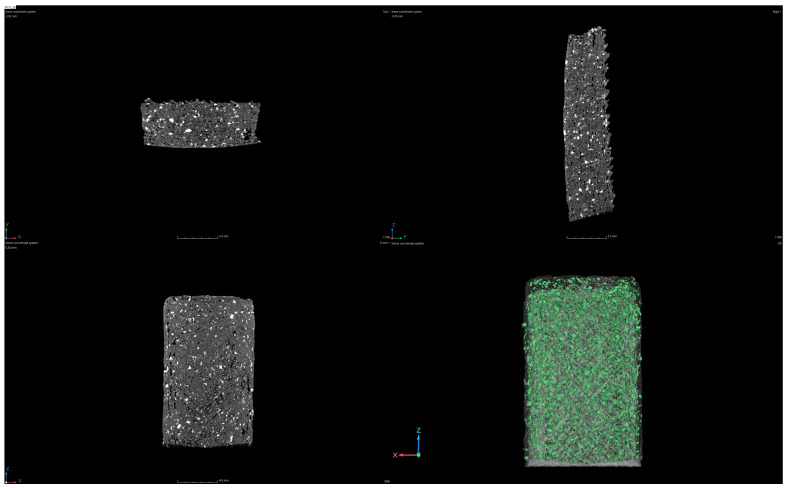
Images from CT for the 3D-printed samples obtained from PP90.
